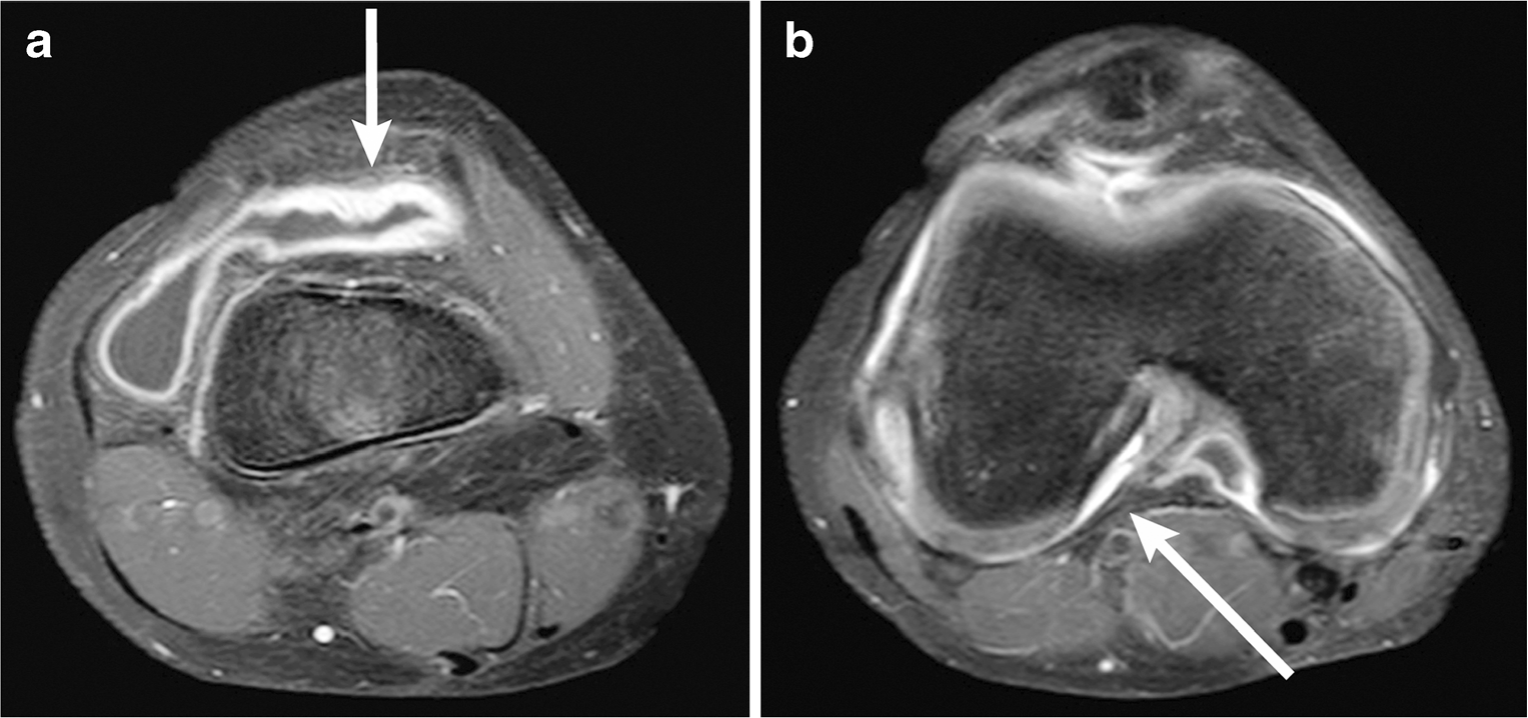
Two examples of how synovial thickness can be measured. Axial T1-weighted fat-saturated MRI images at the suprapatellar region (a) and at the cruciate ligaments (b) of a 10-year old male. In the suprapatellar region (a) contrast shows a synovial thickness of at most 4.1 mm (*arrow*), corresponding to a JAMRIS score of 2 (≥ 4 mm) on this location. At the cruciate ligaments (b) the enhanced synovium is measured at 2.0 mm (*arrow*), corresponding to a JAMRIS score of 1 (≥2 - 4 mm)
